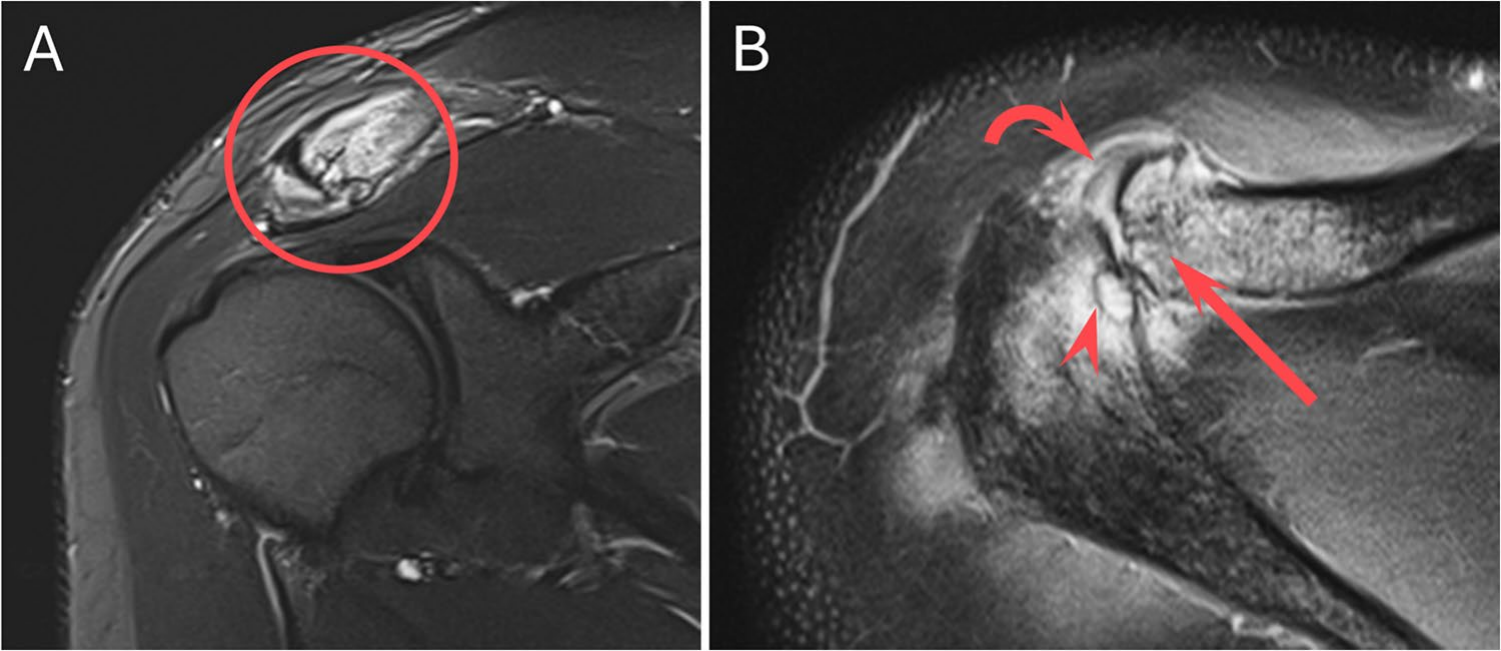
A 28-year-old male presenting a few months after a fall at work. Coronal STIR (A) MR image of the shoulder demonstrates intense bone marrow edema and resorption of the distal clavicle, consistent with DCO (circled). Axial PD fat-suppressed (B) MR image demonstrates similar findings of DCO, including intense bone marrow edema, resorption, and cystic change of the distal clavicle (arrow), capsular thickening and edema (curved arrow), and additional findings of osteolysis involving the anterior acromion (arrowhead). Note the lack of significant bony productive change, which would typically be seen with osteoarthritis
